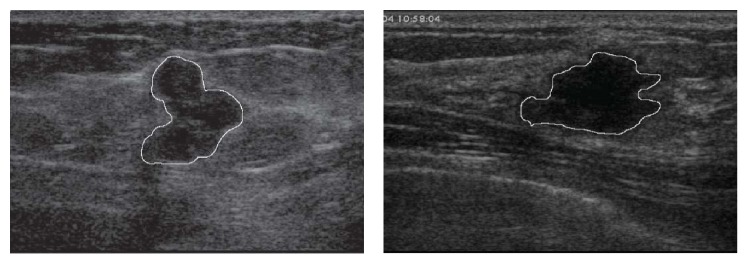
Segmentations of cysts for breast ultrasound image using ANN [32].
